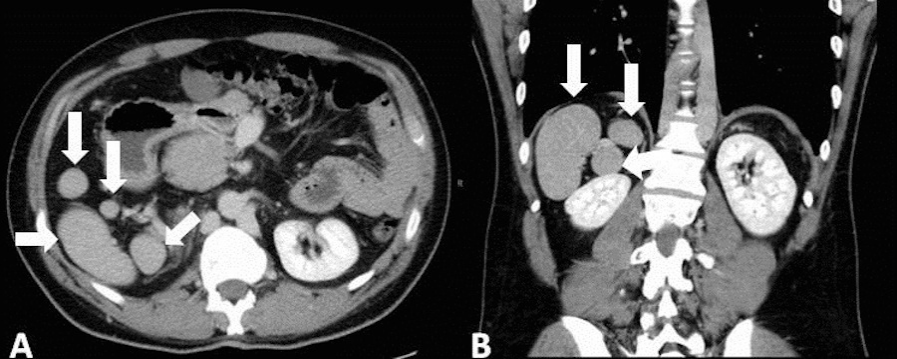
Splenic anatomy by computed tomography Computed tomography of the abdomen from a single patient demonstrating polysplenia. Panel A depicts an axial slice in which multiple well-circumscribed soft tissue masses are identified with attenuation similar to that of a normal spleen in the right posterior abdomen. Panel B depicts a coronal slice which again demonstrates multiple spleens in the right upper abdomen.
